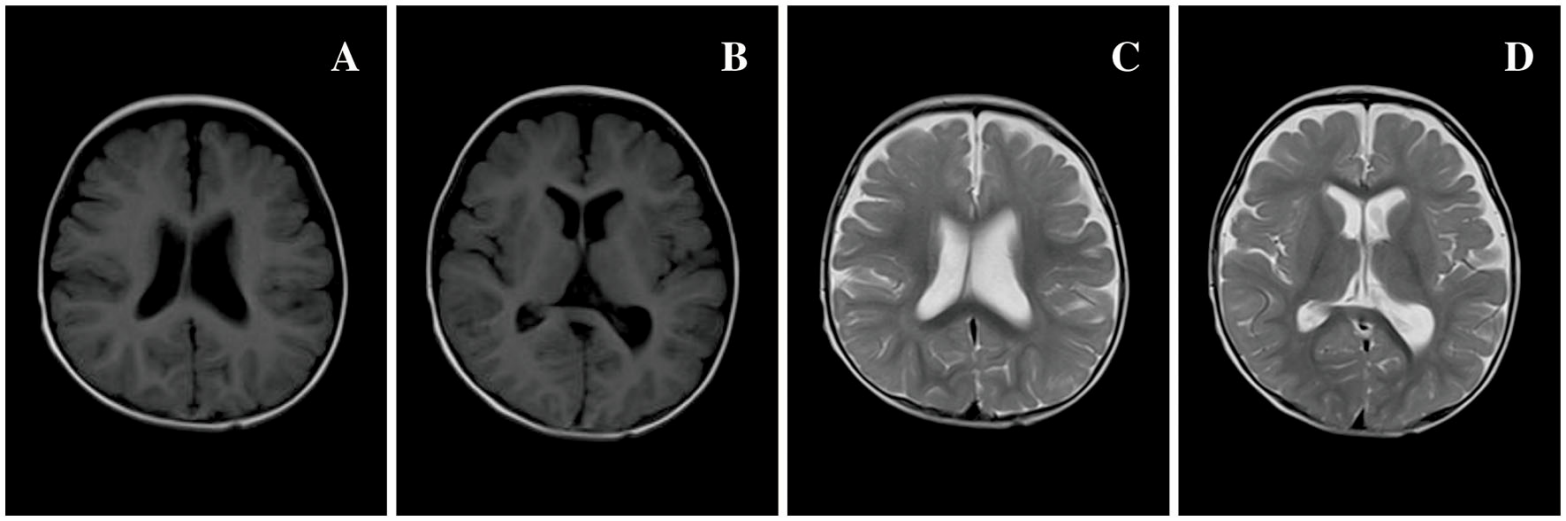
Brain MRI of 80 patients in Table 1 at 7 months of age. (A, B) T1 MRI and (C, D) T2 MRI: bilateral extracerebral spaces of frontal and temporal lobes widened, and the left ventricle was slightly dilated compared to the right side.
